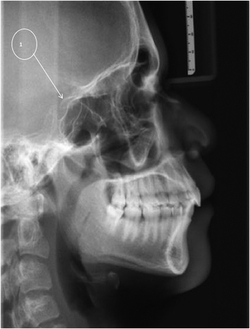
A cephalometric radiograph of DBN 4. Platybasia and a ‘J’ shaped sella turcica are evident (1).
